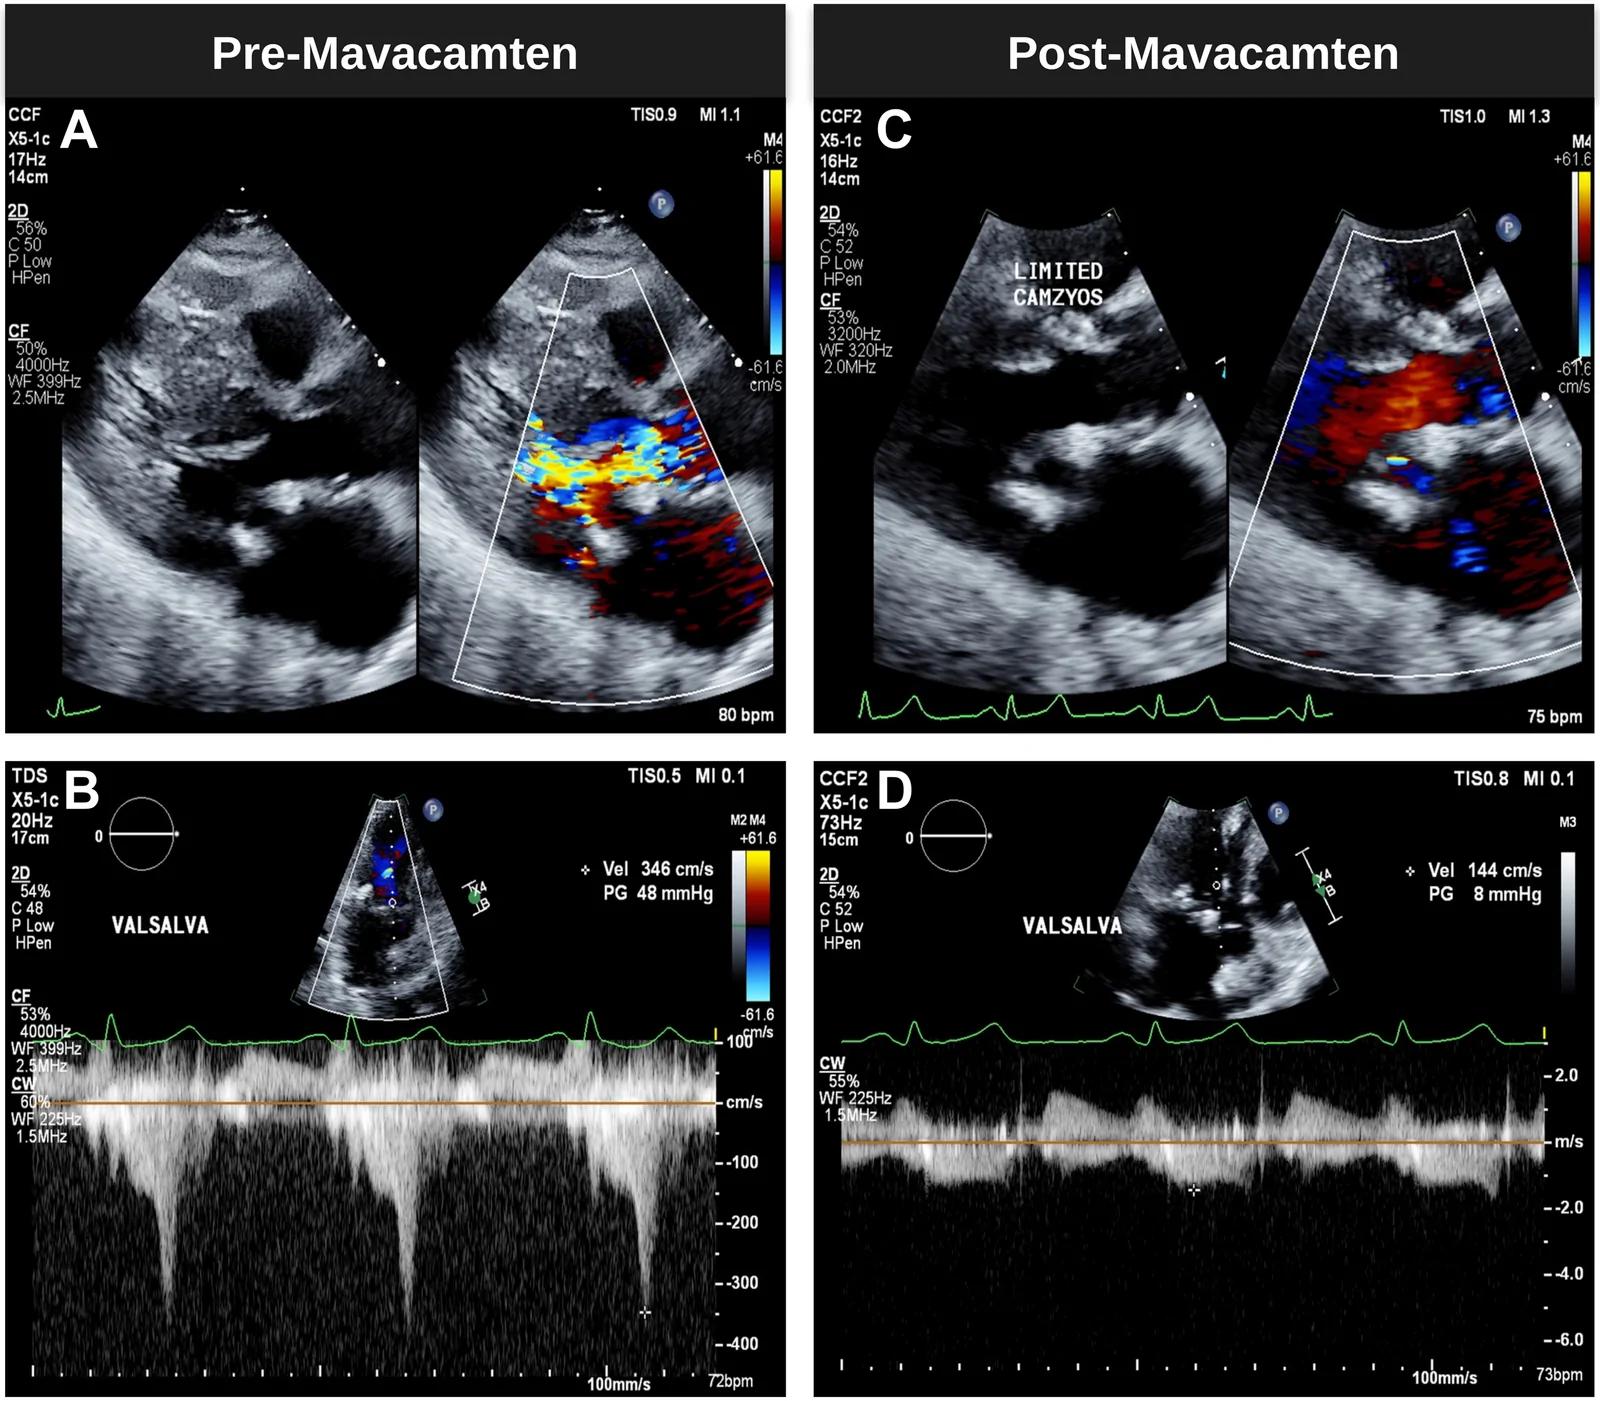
Echocardiographic Assessment Before and After Mavacamten Therapy (A and B) Pretreatment. (A) Parasternal long-axis (PLAX) view with and without color Doppler demonstrating chordal systolic anterior motion (SAM) and left ventricular outflow tract (LVOT) flow acceleration. (B) Continuous-wave (CW) Doppler across the LVOT following Valsalva maneuver, demonstrating an elevated peak gradient (PG 48 mm Hg). (C and D) Post-mavacamten treatment. (C) PLAX view with and without color Doppler showing resolution of chordal SAM and reduction in LVOT obstruction. (D) CW Doppler across the LVOT following Valsalva maneuver demonstrating marked reduction in peak gradient after mavacamten therapy (PG 8 mm Hg).
